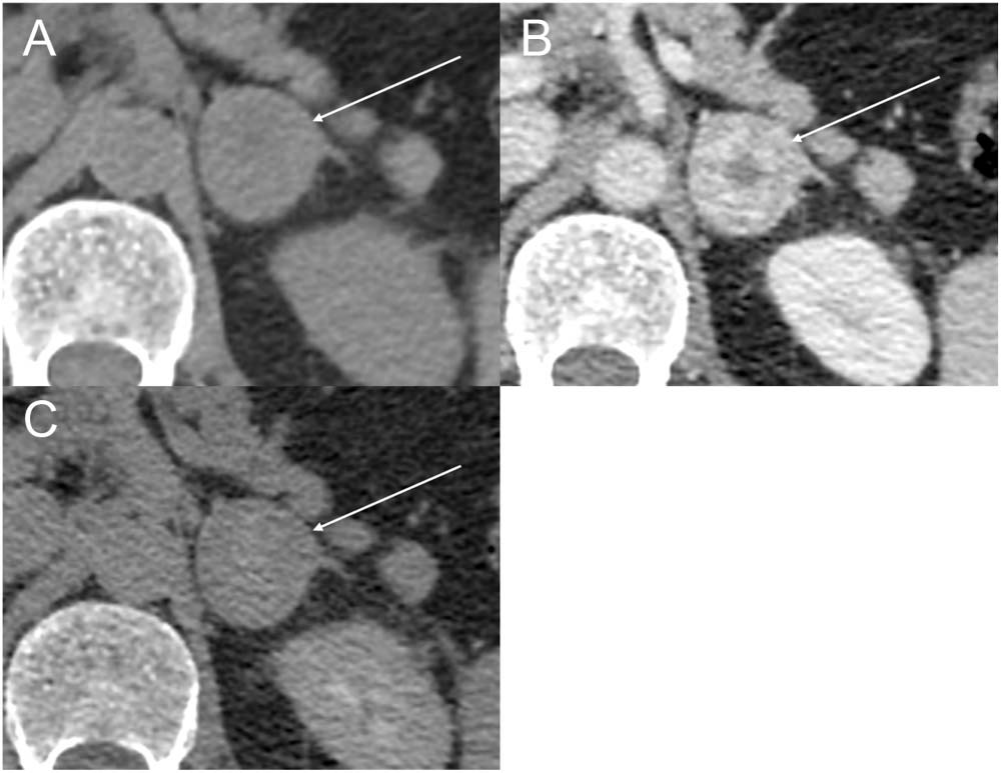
Axial CT images of a left 3.2 cm adrenocortical carcinoma. (A) Unenhanced CT demonstrates a lesion with a mean attenuation of 26 Hounsfield units (HU). (B) Contrast-enhanced CT at 75 s post-intravenous contrast administration shows heterogeneous enhancement, with a peak attenuation of 79 HU. (C) Delayed-phase CT at 10 min demonstrates near-complete washout, with an attenuation of 27 HU. The lesion’s heterogeneous enhancement and incomplete contrast washout are characteristic of malignant adrenal neoplasms, distinguishing it from benign adenomas.
